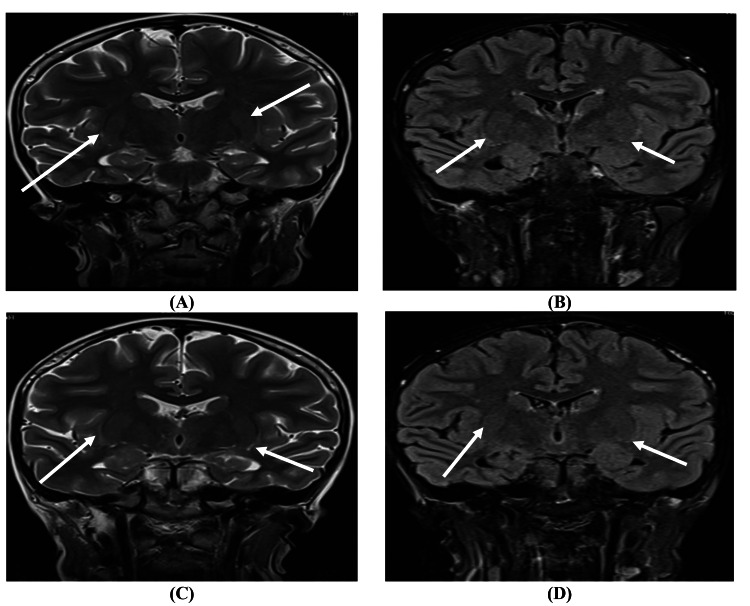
(A, C) Coronal T2-weighted imaging sequence. (B, D) Coronal FLAIR sequence showing bilateral external capsule/claustrum hyperintensities in coronal T2/FLAIR T2/FLAIR: T2-weighted/fluid-attenuated inversion recovery
